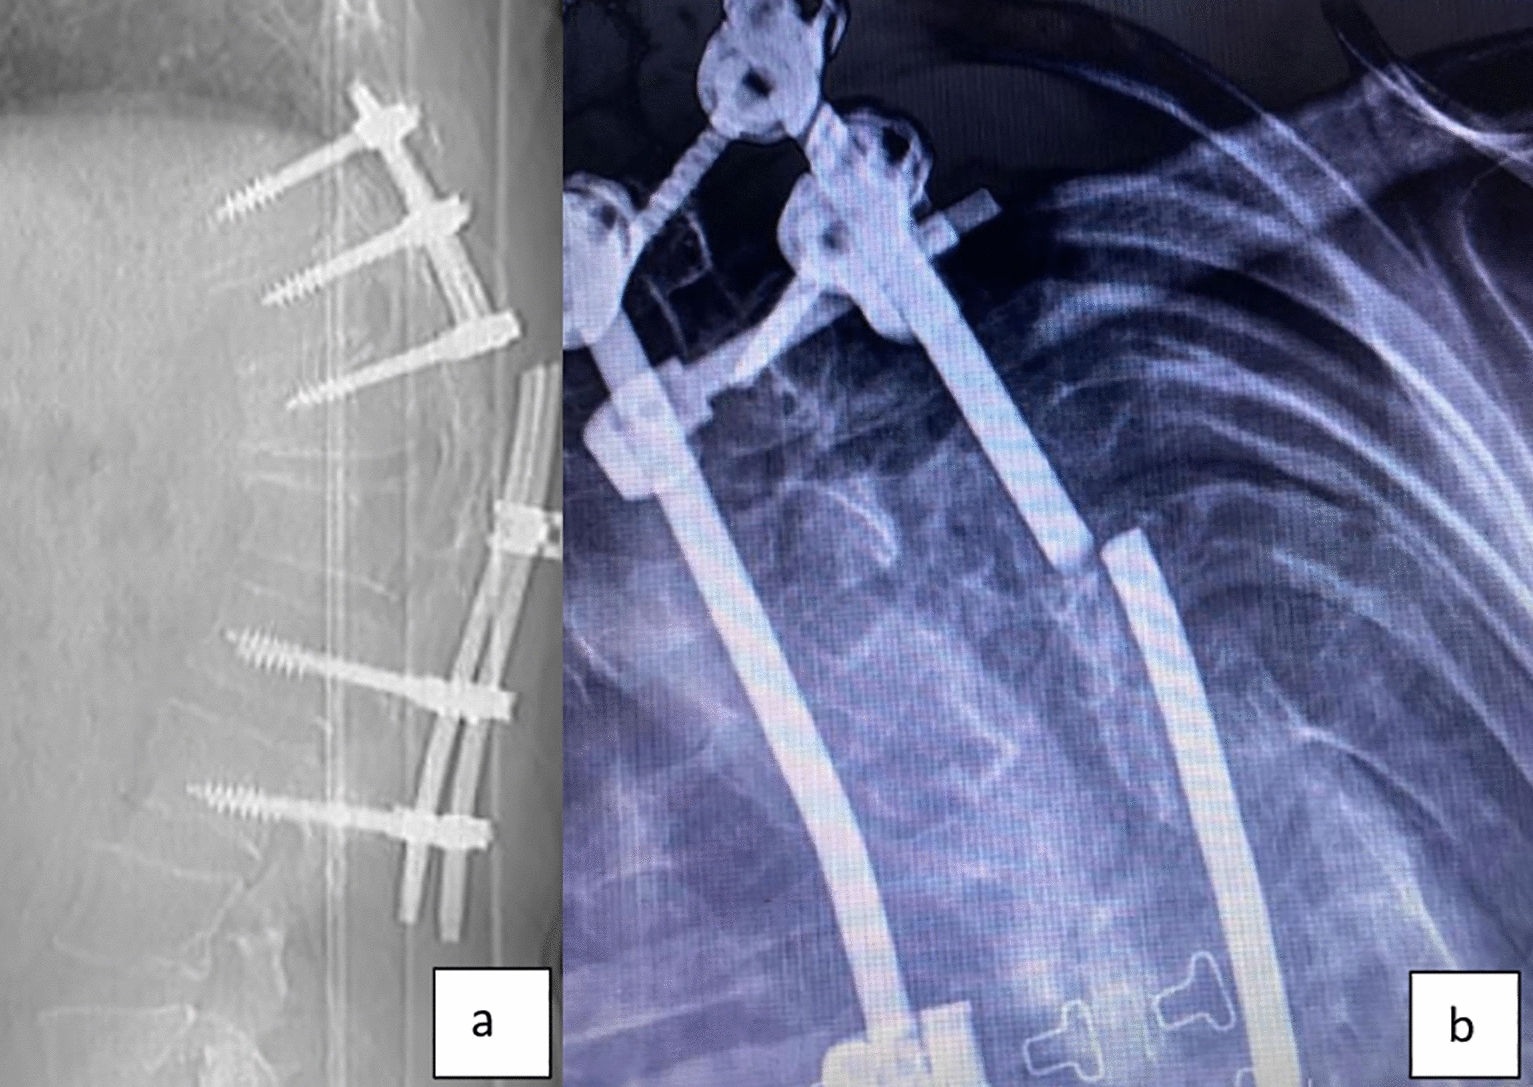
a, b Lateral and anteroposterior X-ray images demonstrating rod breakage
